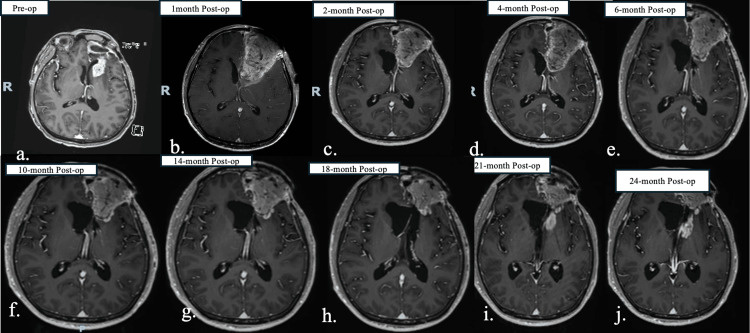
T1 contrast-weighted serial MRI T1 contrast-weighted MRI showing a) pre-operative recurrent left frontal tumor; b) post-operative status with gross total resection of the left frontal tumor with omental cranial transposition without any evidence of disease; c-h) serial follow-up imaging shows stable imaging with progressive shrinkage of the omental flap; i) imaging at the 21st month showing recurrent disease at the left frontal operative site with further shrinkage of the omental flap; j) imaging at the 24th month showing slowly progressive disease.
